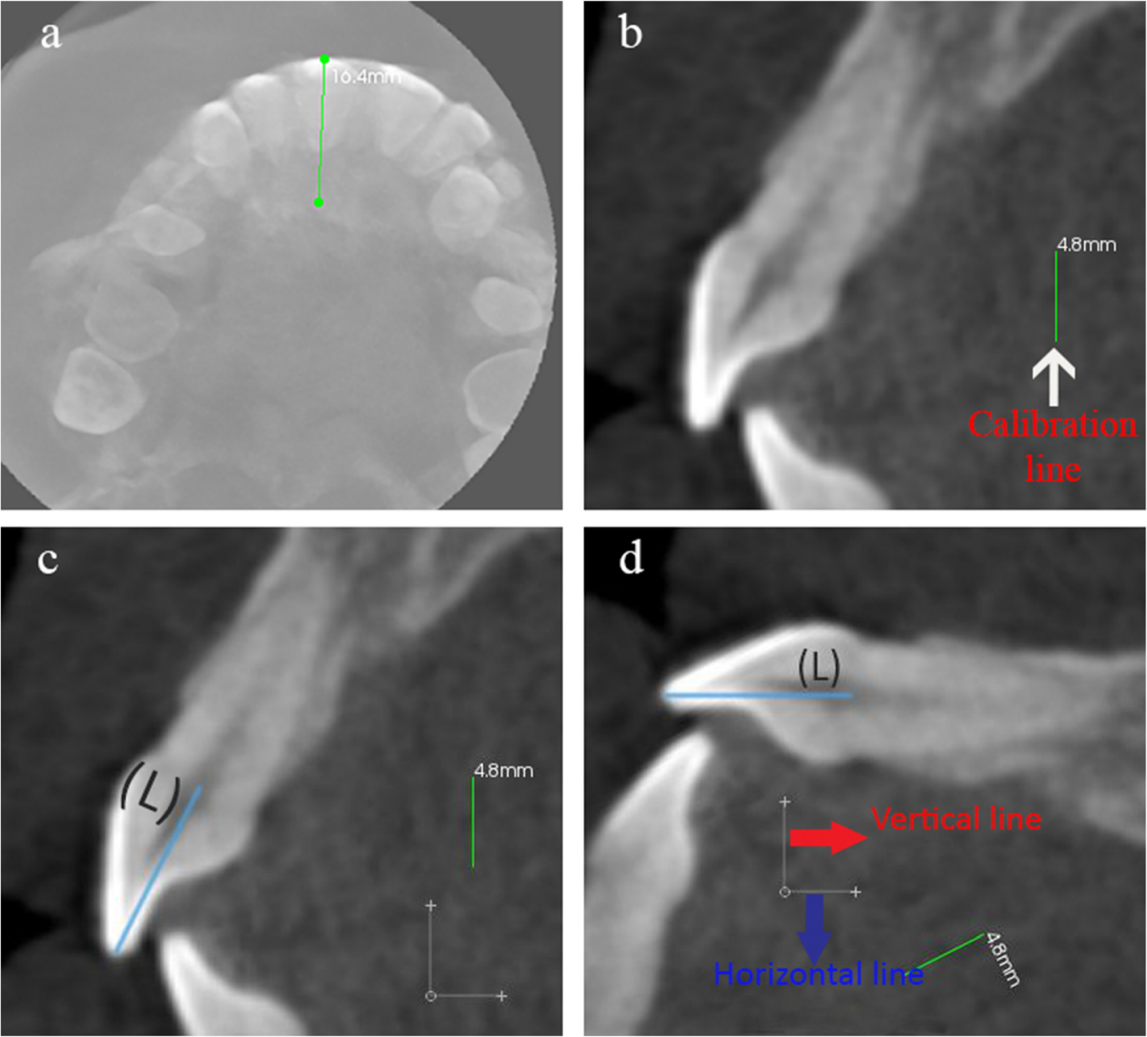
a Labio-palatal axis of RMCI (drawn line) was crossed by the sagittal plane indicator in the axial slice of CBCT image. b Sagittal slice of CBCT image showing proximal aspect of RMCI and calibration line. c The red line joins palatal and labial CEJs, while blue line (L) represents crown’s long axis. d Image rotation was done such that line (L) became horizontal
